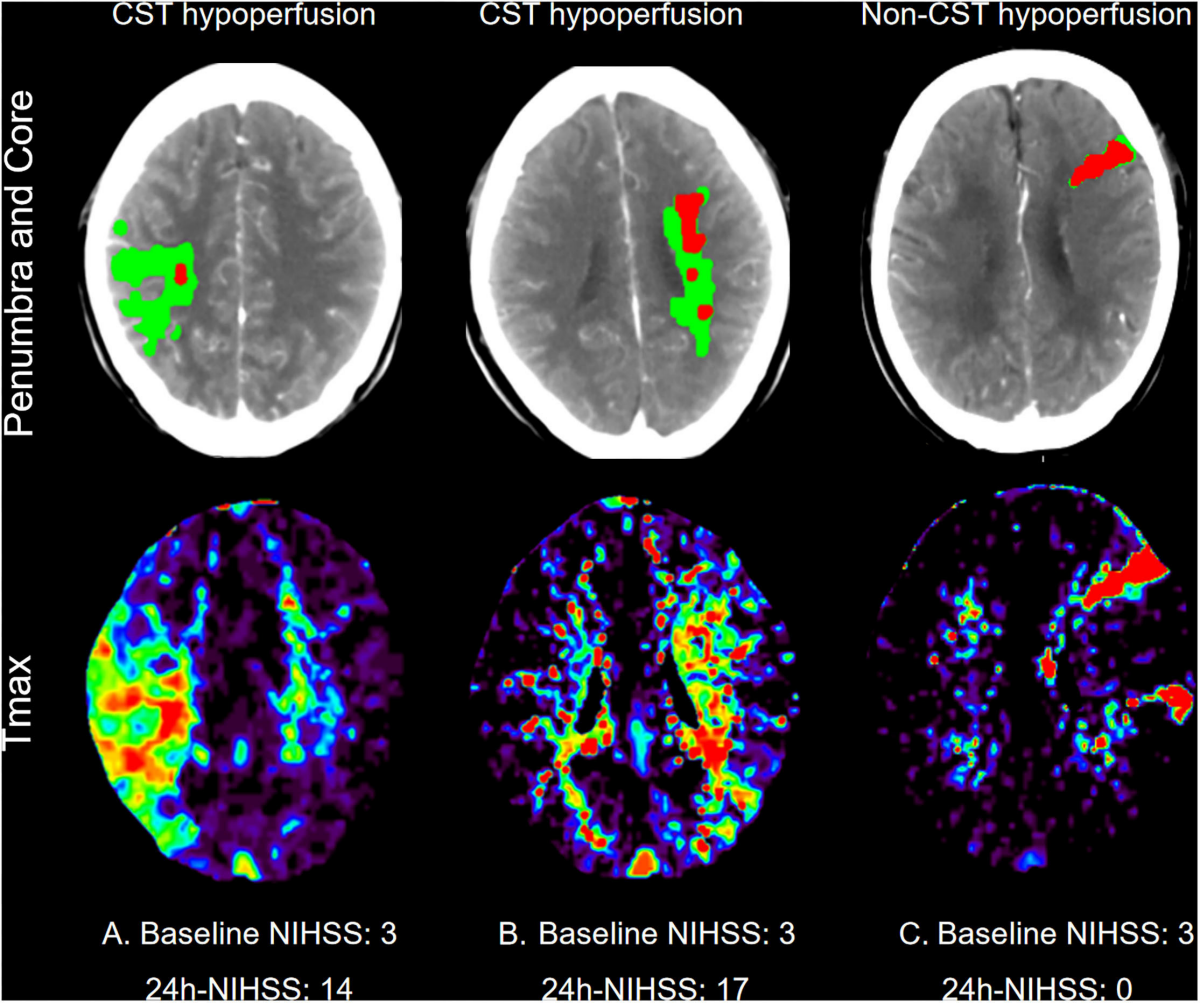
Representative examples of patients who underwent computed tomography perfusion at baseline. First line: Penumbra (Green Area) and core (Red Areas) Map; Second line: Time to maximum of tissue residue function (Tmax) map. (A) One patient with CST hypoperfusion at right corona radiata, baseline NIHSS: 3; 24h-NIHSS: 14. (B) One patient with CST hypoperfusion at left lateral ventricle, baseline NIHSS: 3; 24h-NIHSS:17. (C) One patient with non-CST hypoperfusion, baseline NIHSS: 3; 24h-NIHSS: 0.
